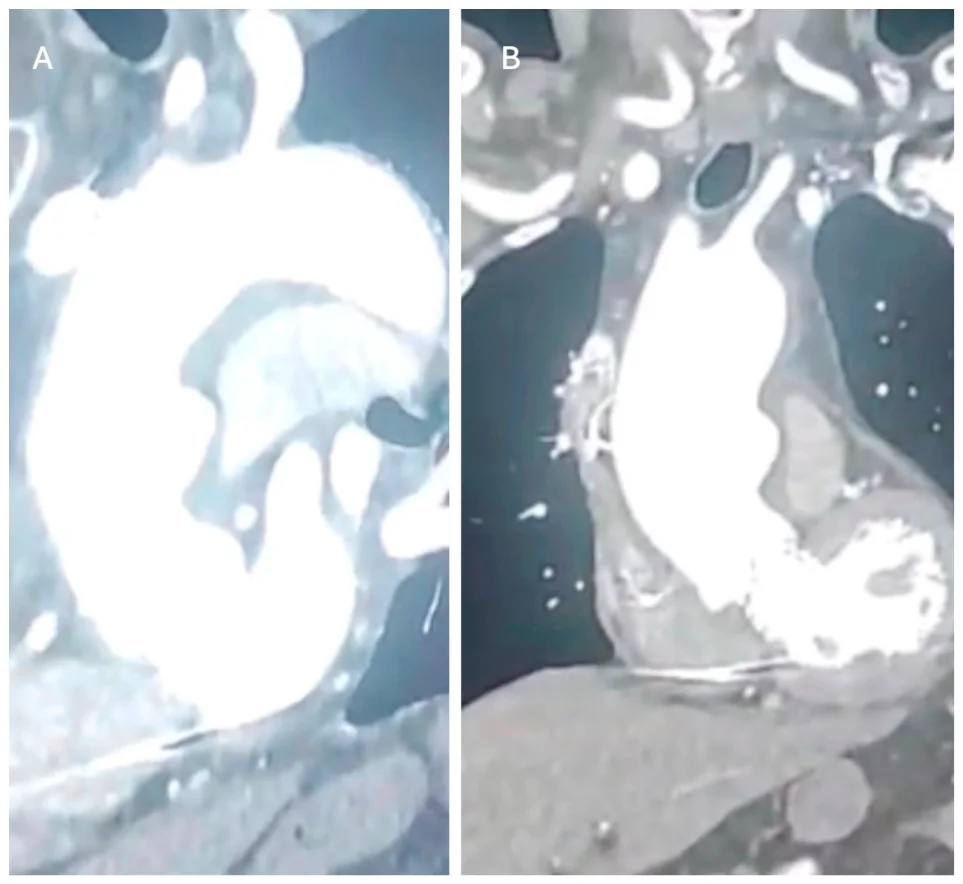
(A,B). Computed tomography angiography revealed Stanford type A acute aortic dissection involving the ascending aorta.
